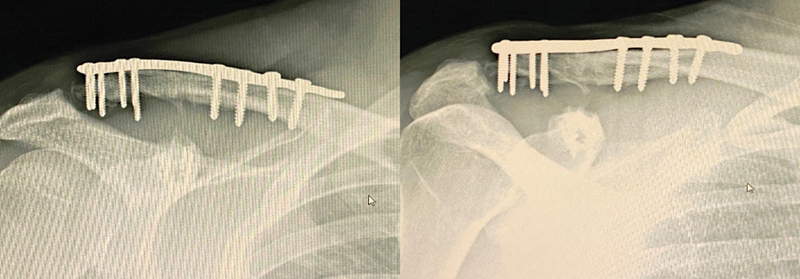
Radiograph 12 months after surgery showing fracture consolidation and no signs of osteonecrosis.
